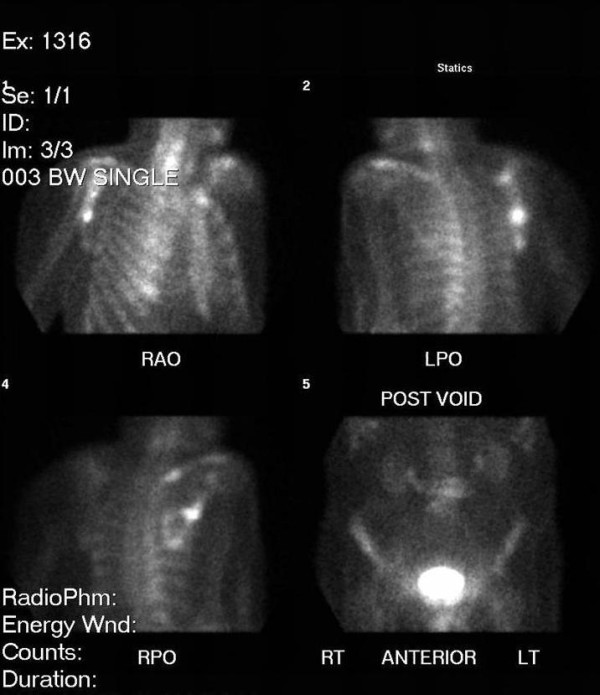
Bone scintigraphy of the right scapula reveals increased uptake where metastatic lung carcinoma is present.
